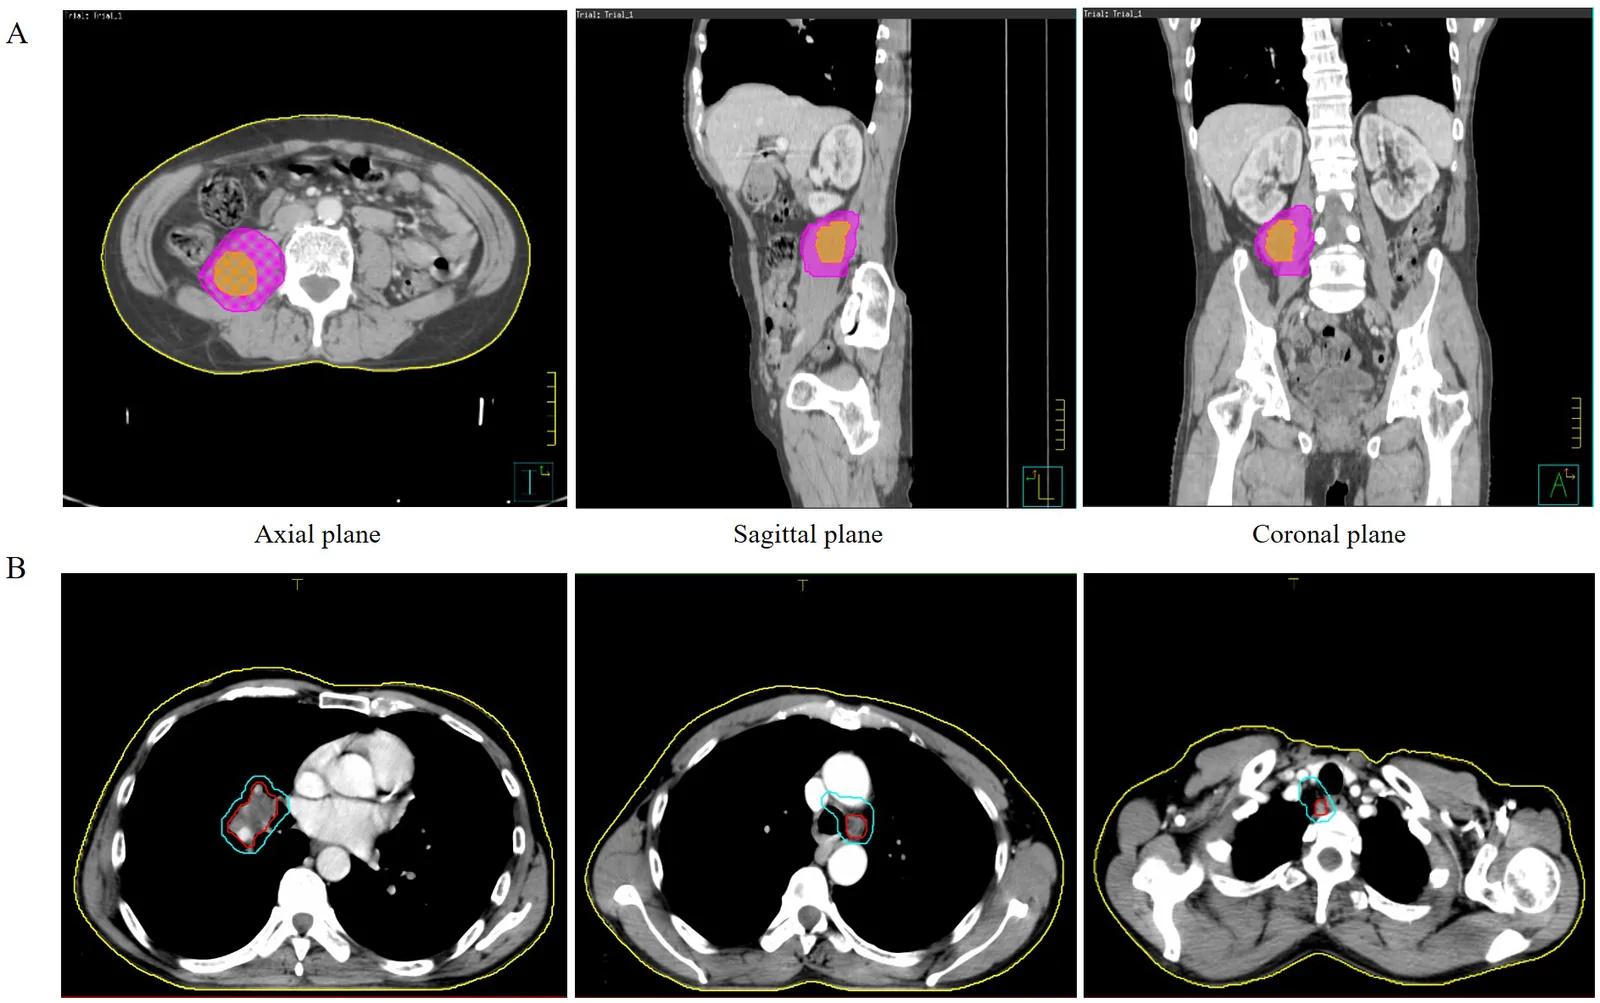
Radiotherapy target volume. (A) Radiotherapy target volume for a right-sided psoas major muscle metastasis. The dose was 75 Gy in the yellow part (GTV-core: necrosis area), 50 Gy in the purple part (pGTV: radiographically visible right psoas major muscle metastasis). (B) Radiotherapy target volume for the right hilar tumor and mediastinal metastatic lymph nodes. The dose was 60 Gy in the red part (GTV: radiographically apparent tumor), 54 Gy in the blue part (PTV: Planning target volume).
